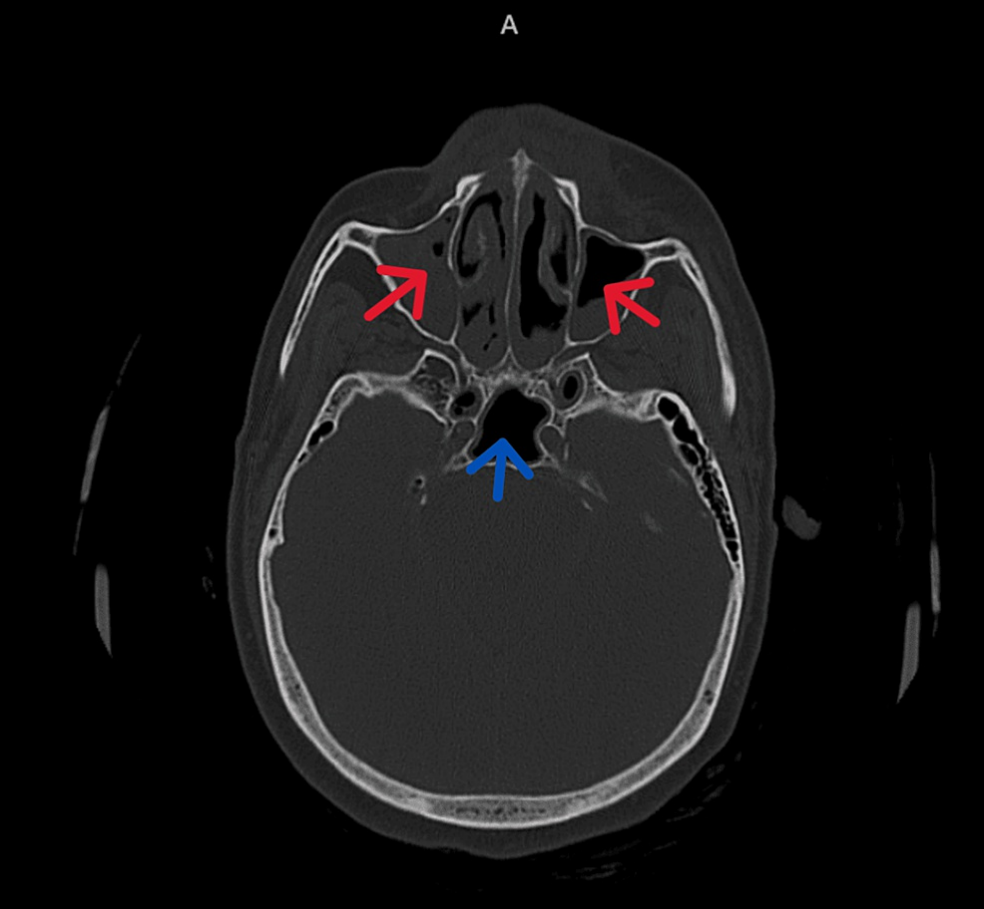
Non-contrast CT (NCCT) brain (bone window) showing maxillary (red arrow) and sphenoidal sinuses (blue arrow) Near complete opacification of right maxillary sinus with mild sclerosis of the bony walls. Mucosal thickening of left maxillary sinus and bilateral sphenoid air cells.
